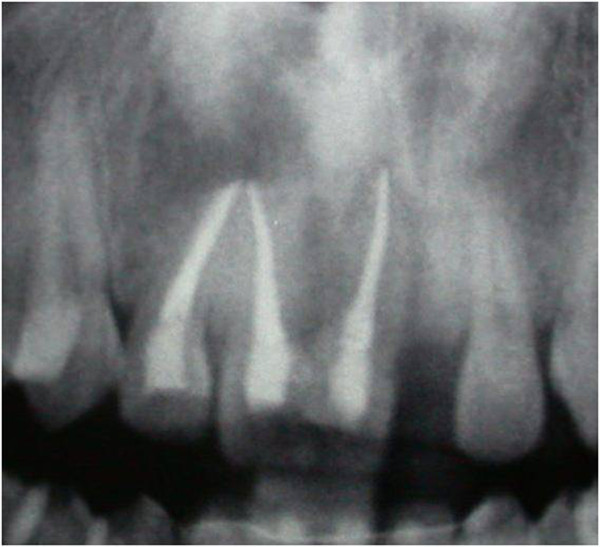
Radiographic control at six months.
